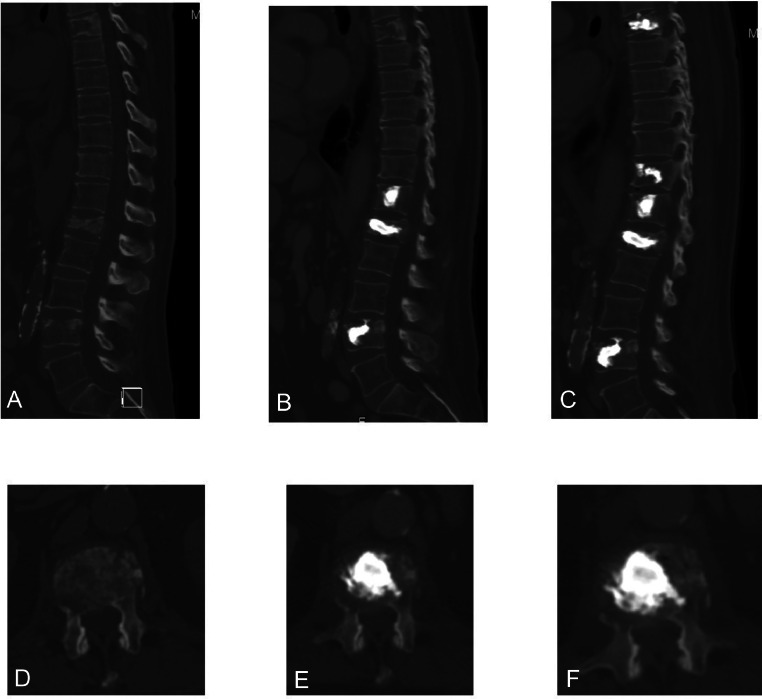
Sagittal (A, B, and C) and axial (D, E, F) showing CT scan findings at presentation (A and D), 2 weeks after surgery (B and E) and 6 weeks after surgery (C and F).
